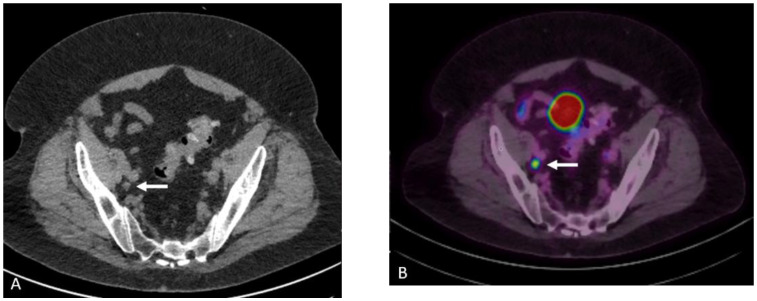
Seventy-seven-year-old male with history of prostate cancer, status post-prostatectomy, now with biochemical recurrence. NECT (A) and corresponding fused PET/CT (B) images show a sub-centimeter right external iliac lymph node which shows moderate radiotracer uptake concerning for nodal metastatic disease.
